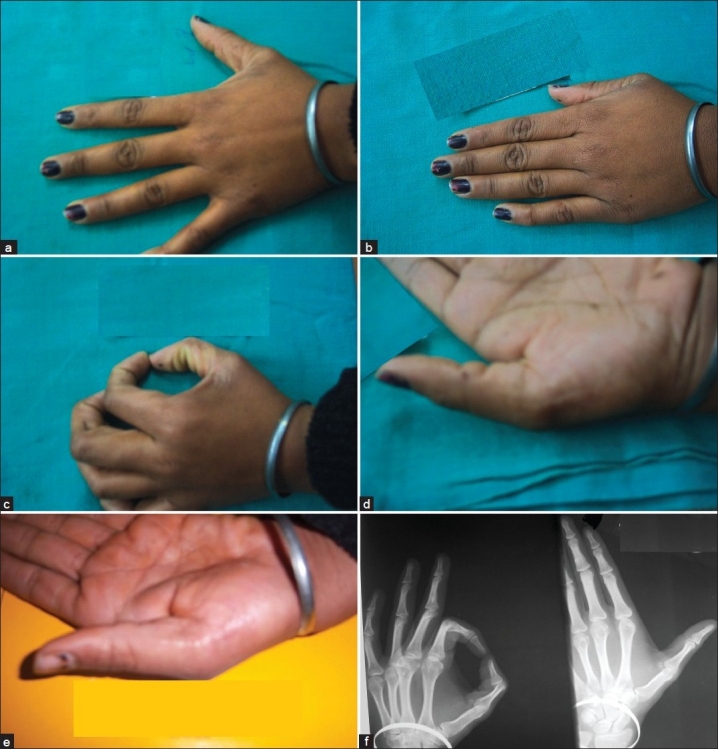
(a-e) Clinical photograph of left hand at 3 years follow-up showing old healed surgical scar on radial aspect of first metacarpal, without any bulge in the first space and normal range of motion of the left thumb (Figure 1 and  2 a-e). (f) Final radiograph at end of 3 years, showing healed excised part of bifid first metacarpal and normal interphalangeal and metacarpophalangeal joints
